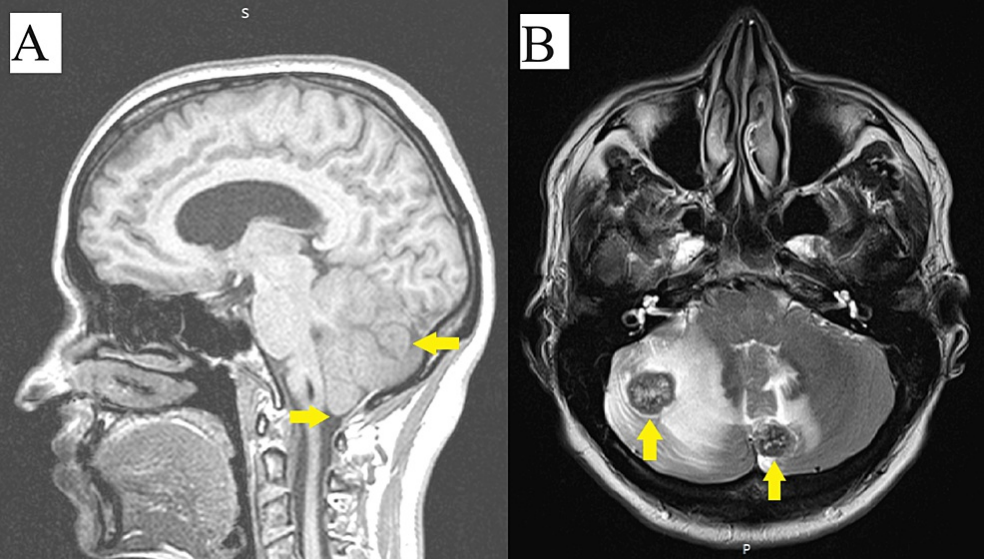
MRI scan. (A) the right yellow arrow showed cerebellar metastasis and the left yellow arrow a slight herniation of cerebral tonsils through the foramen magnum – sagittal section T1 and (B) two cerebellar metastases with perilesional edema – transverse section T2.
